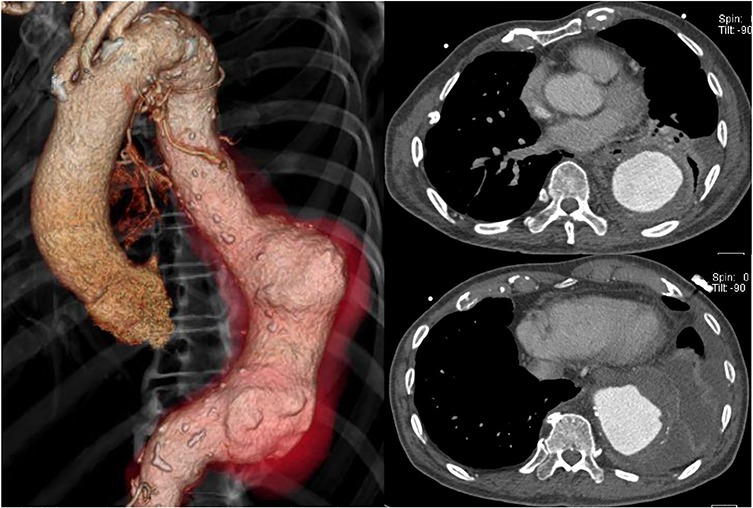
Preoperative CT shows acute expansion of the aneurysm to 70 mm in diameter.
